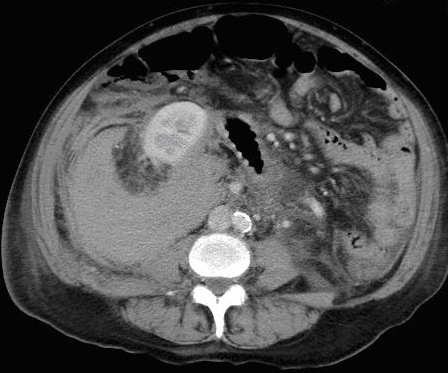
Axial CT image demonstrates a large right retroperitoneal haematoma surrounding the angiomyolipoma and displacing the right kidney anteriorly.
